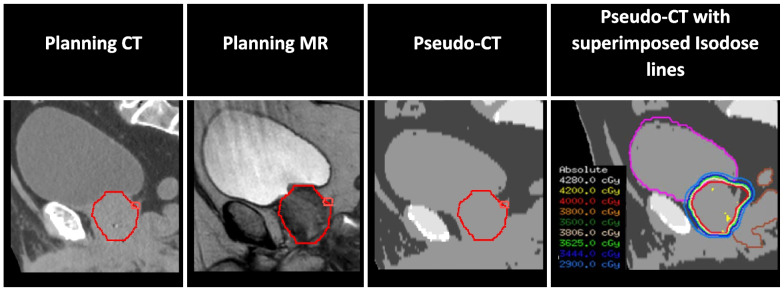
The MR-planning was used to generate a pseudo-CT for treatment planning and dose calculation (red = prostate; pink = seminal vesicles; magenta = bladder; brown = rectum)
